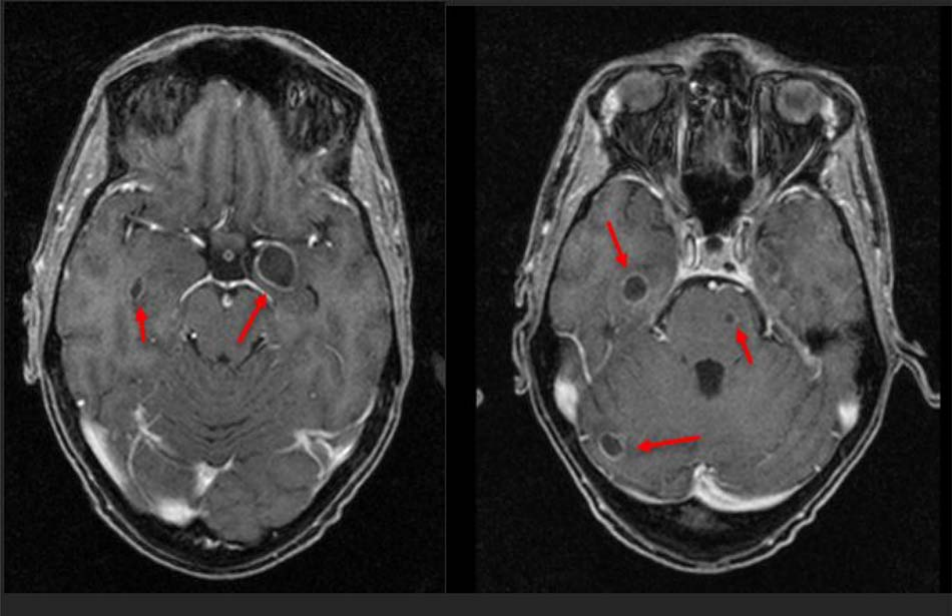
First MRI scan showing deeper brain abscesses.
